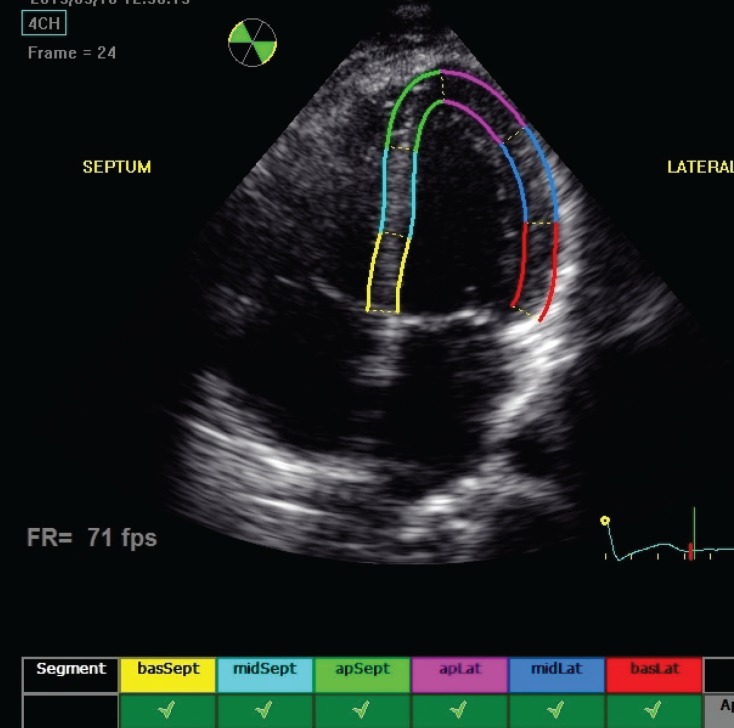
Echocardiograph shows segmental analysis of left ventricle after 2D speckle tracking from the apical fourchamber view.
